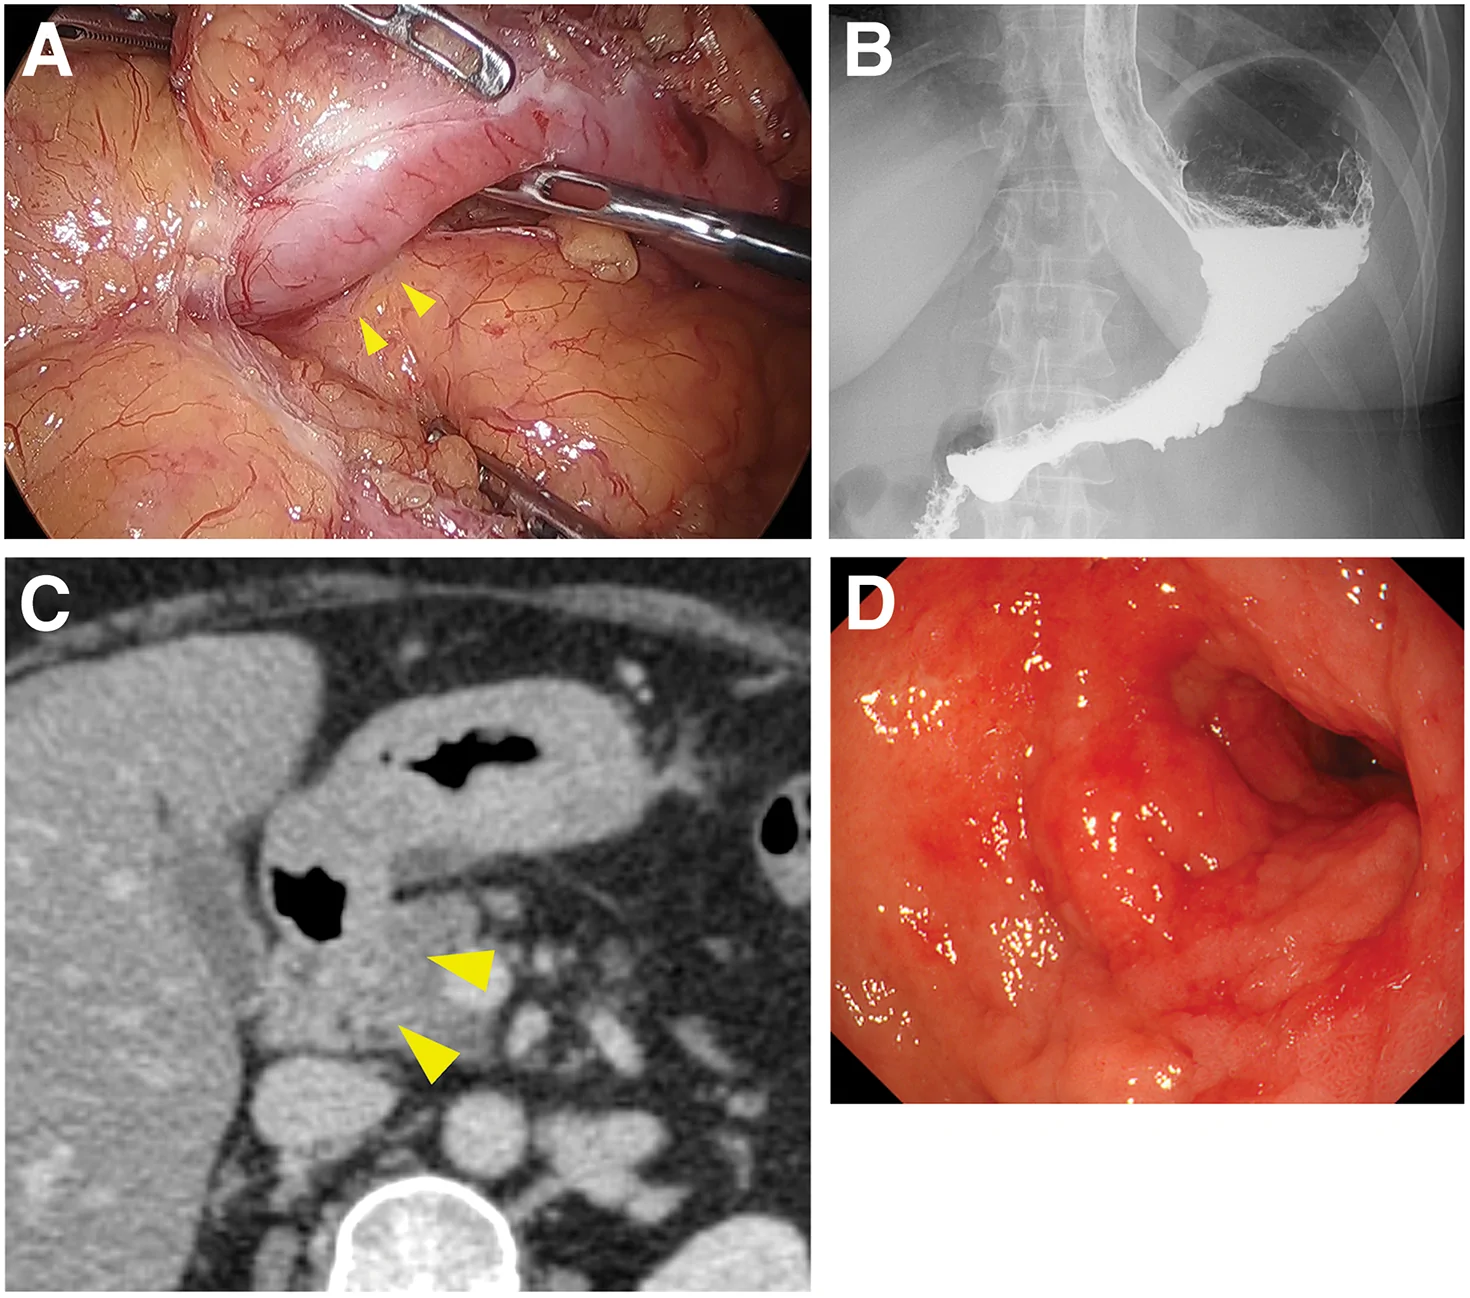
Pre-treatment findings. (A) Diagnostic laparoscopy demonstrated direct pancreatic invasion (arrow). (B) Upper gastrointestinal series demonstrated diffuse wall thickening and marked rigidity of the lower gastric body with poor distensibility. The gastric lumen appeared narrowed without a distinct mass lesion, findings consistent with type 4 gastric cancer. (C) Contrast-enhanced CT before chemotherapy demonstrating direct pancreatic invasion (arrowheads). (D) Upper gastrointestinal endoscopy before chemotherapy showing diffuse wall thickening and poor distensibility of the lower gastric body, consistent with type 4 gastric cancer.
